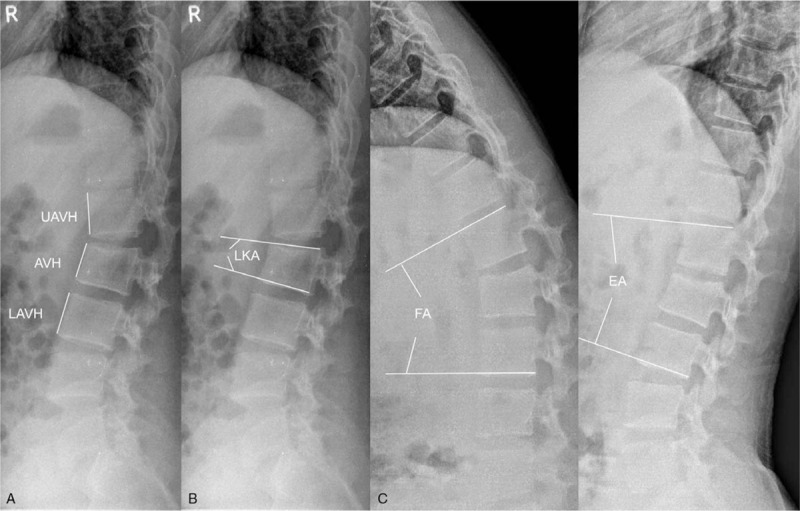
Plane radiographs of an 18-year-old man with an L2 burst fracture. Standing lateral image after removal surgery showing the (A) ABHR and (B) LKA; ABHR = AVH/(UAVH + LAVH)/2, LKA: the angle between the superior and inferior end plate of the fractured vertebra. (C) By 10 years after removal surgery, we determined the inter-segmental motion angle (flexion angle subtracted from the extension angle) by Cobb angle method. AVH = anterior vertebral body height of the fractured vertebra, LAVH = lower anterior vertebral body height, UAVH = upper anterior vertebral body height, FA = flexion angle, EA = extension angle.
